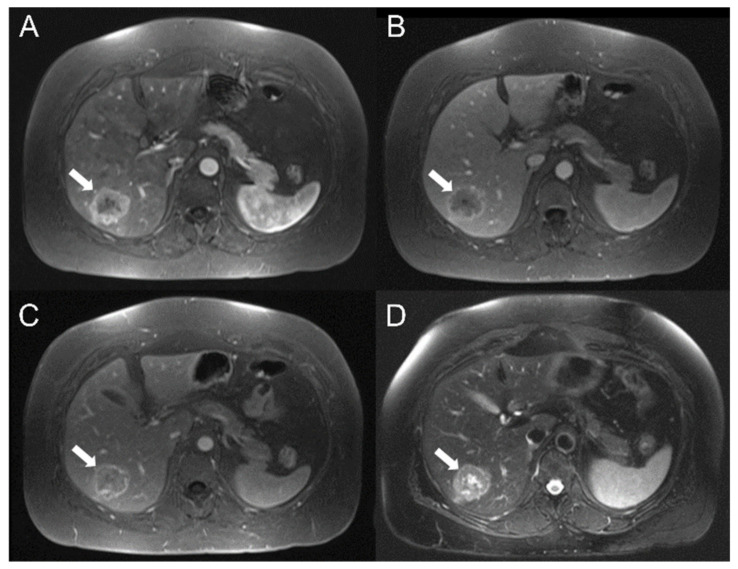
Moderately differentiated HCC (G2) in the right hepatic lobe of a 54-year-old white female. Results showed hypointense mass on axial dynamic study (A–C), and hyperintensity on the lesion central part on fat-suppressed axial T2-weighted sequence (D) (white arrow).
